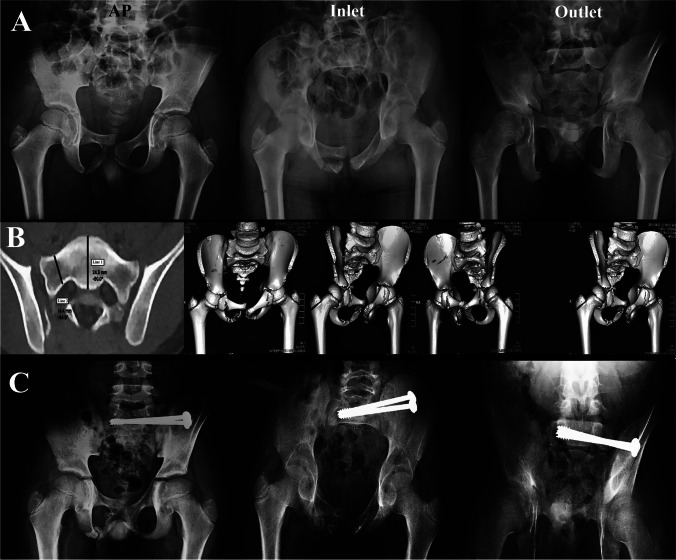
Male child 10 years old presented after a motor car accident with fracture pelvis type C1.2 (with Lt sacroiliac dislocation) treated by iliosacral screws. A Preoperative plain radiographs. B Preoperative CT scan showing how to plan the size of screws to be used for fixation. C Plain radiographs after 24 months of follow-up
